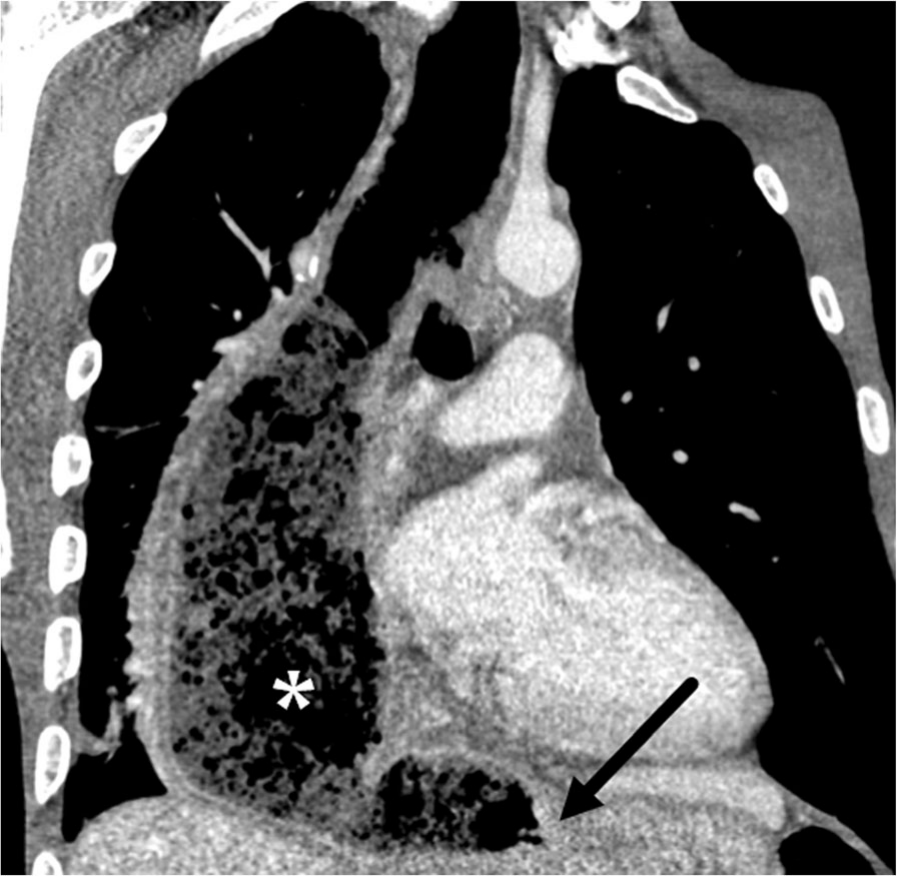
Primary achalasia. Forty-eight-year-old male with dysphagia and weight loss. Coronal CT image shows markedly dilated esophagus with an air-debris level (asterisk). An abrupt stricture (arrow) is seen in the lower esophagus (bird beak sign)
